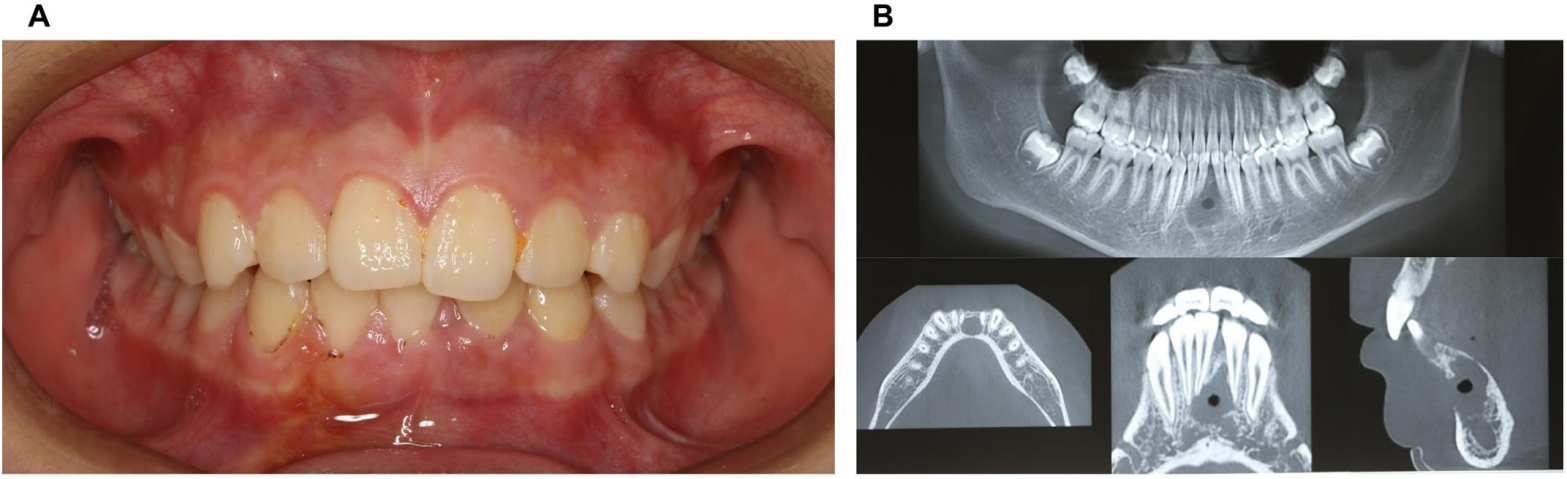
Physical and CBCT examination two weeks after surgery. (A) Photos of intraoral view; (B) CBCT examination.
